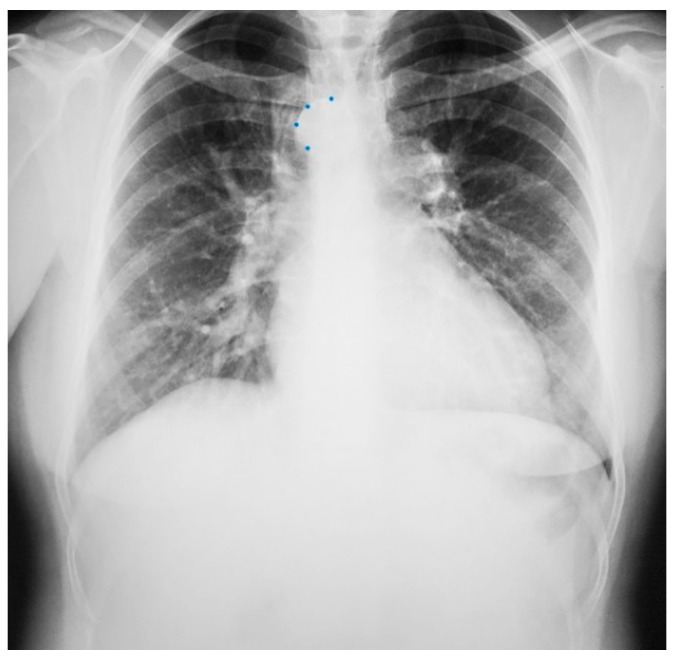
Posteroanterior chest X-ray of sinus venosus atrial septal defect. This chest X-ray is of a 24-year-old female with a sinus venosus atrial septal defect and anomalous right upper pulmonary venous drainage to the superior vena cava. The image is well centered and there is a good inspiration. The cardiothoracic ratio is increased, the pulmonary artery segment is increased and the pulmonary vascularity is plethoric. Also note that there is a right-sided aortic arch (blue dots). This allows the enlarged pulmonary trunk to be better visualized because the thoracic aorta does not obscure it. Because she had dyspnea, fatigue and exercise intolerance she underwent surgical repair of the ASD and redirection of the right upper pulmonary vein to the left atrium. Her postoperative CXR showed decrease in C-T ratio and pulmonary vascularity. Her symptoms resolved.
